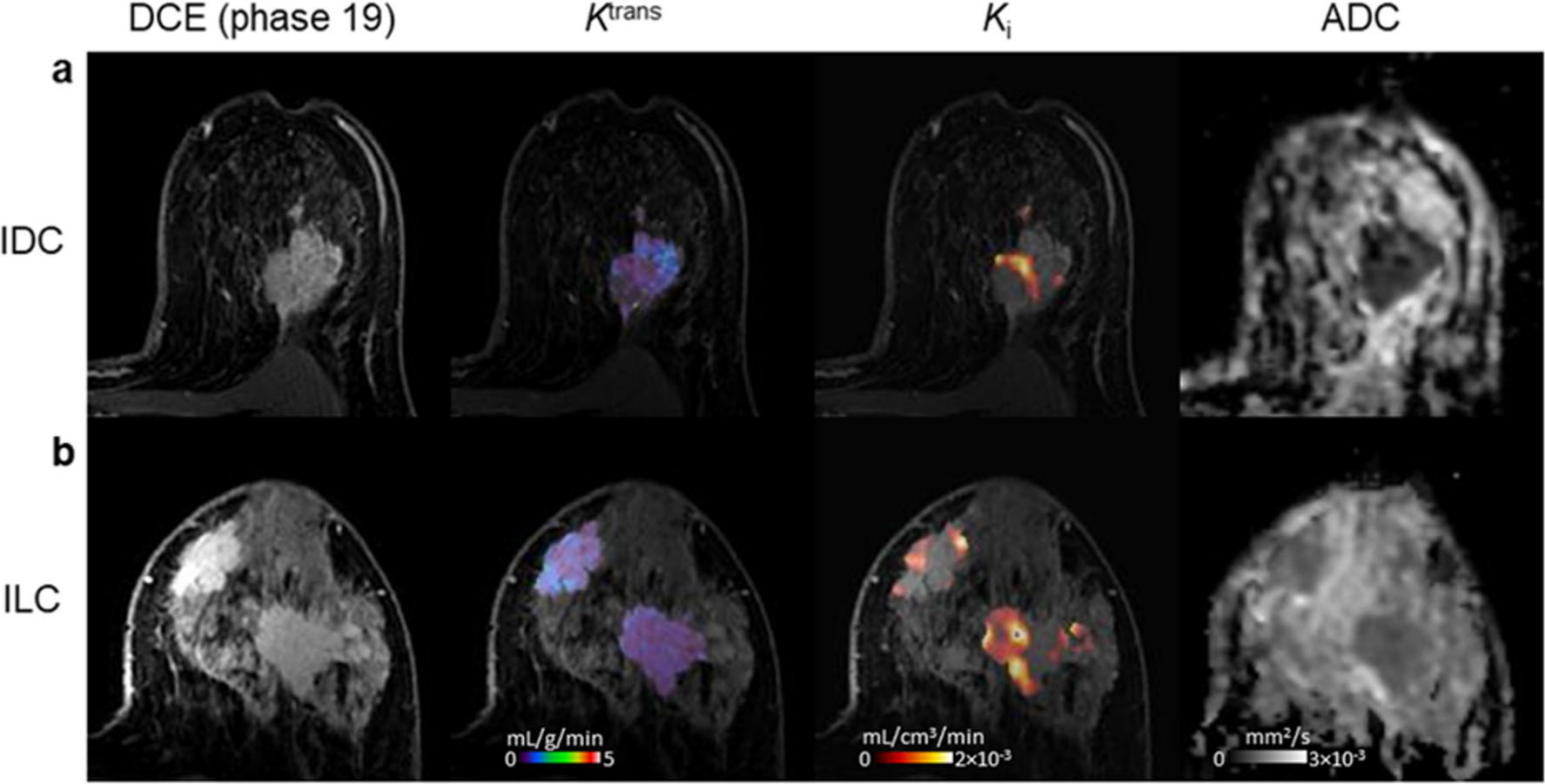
Axial images of two patients with: a invasive ductal carcinoma (IDC); b invasive lobular carcinoma (ILC). (*Left-to-right*) DCE-MRI image at peak enhancement; *K*^trans^ map representing tumour perfusion for the lesion ROI overlaid on the peak-enhancing DCE-MRI image; *K*_i_ map representing tumour hypoxia for the lesion ROI overlaid on the DCE-MRI image at peak enhancement; ADC map. *K*^trans^, contrast influx rate (mL/g/min); *K*_i_, ^18^F-FMISO influx rate (mL/cm^3^/min); ADC, apparent diffusion coefficient (mm^2^/s)
